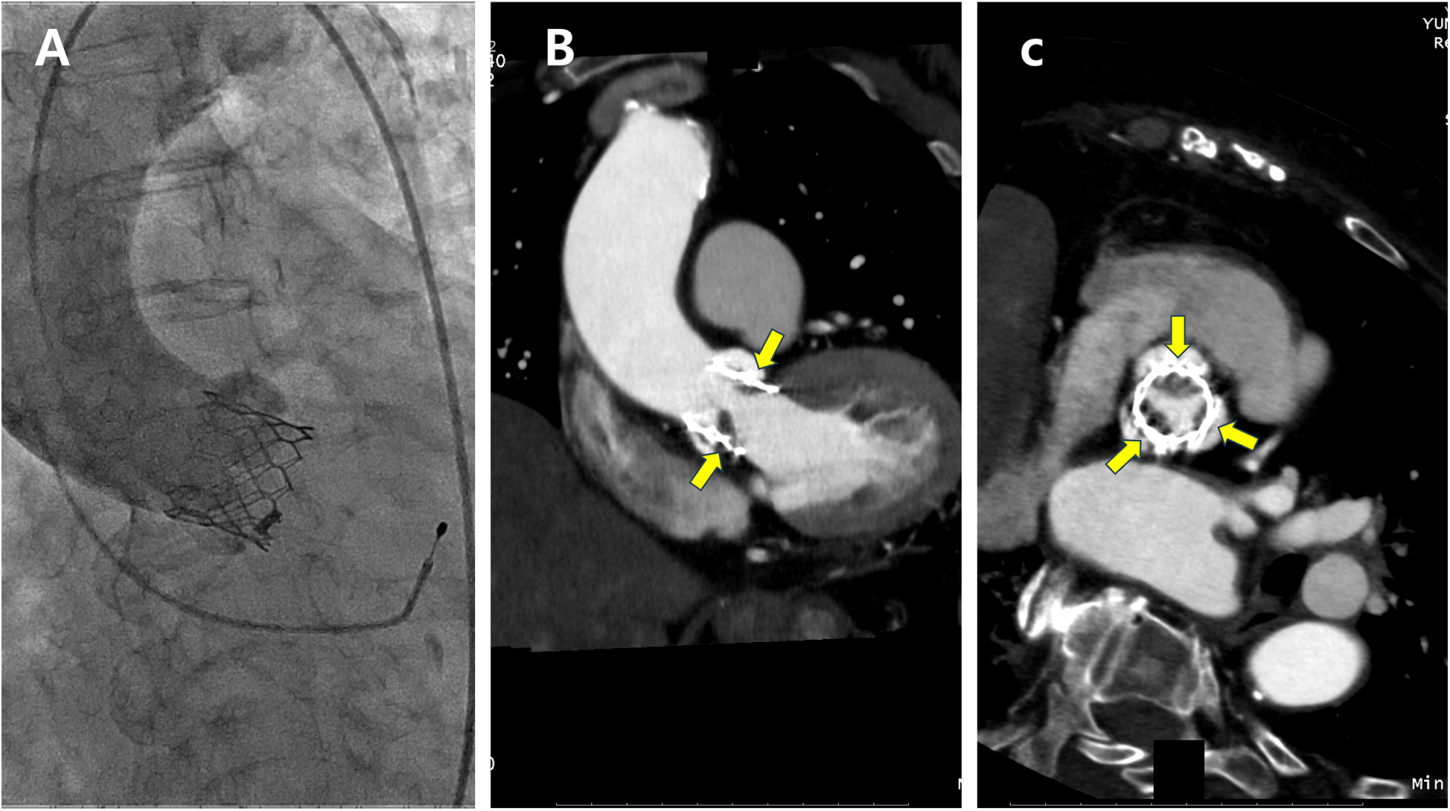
A patient with subclinical leaflet thrombosis detected on computed tomography (CT) at 3-month follow-up. (A) Aortography immediately after transcatheter aortic valve replacement. (B,C) Implanted aortic valve with hypoattenuated leaflet thickening (yellow arrows) on CT suggestive of leaflet thrombosis (B, longitudinal view; C, short axis view).
